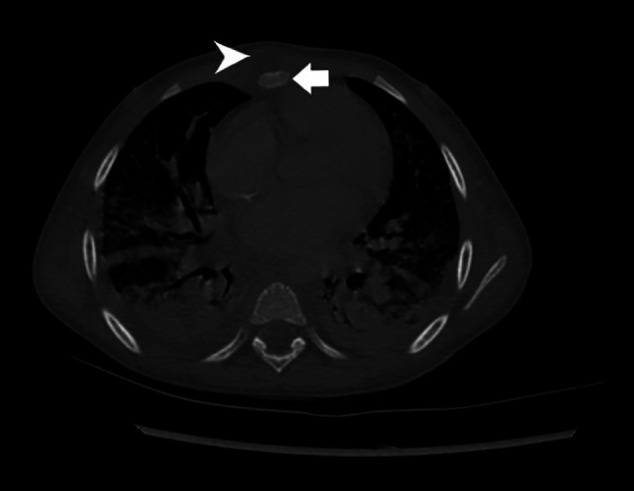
Chest computed tomography. White arrow indicates osteomyelitis of the body of the sternum. White arrowhead indicates thoracic abscess anterior to the sternum. Consolidation caused by multifocal pneumonia is also evident.
